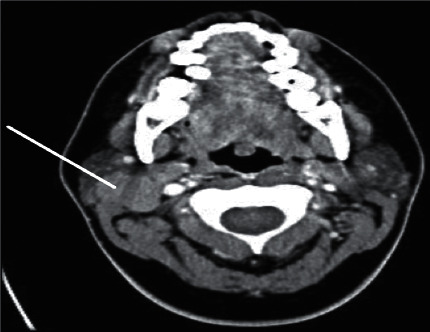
The CT scan showing a right parotid gland lesion in 2017.
